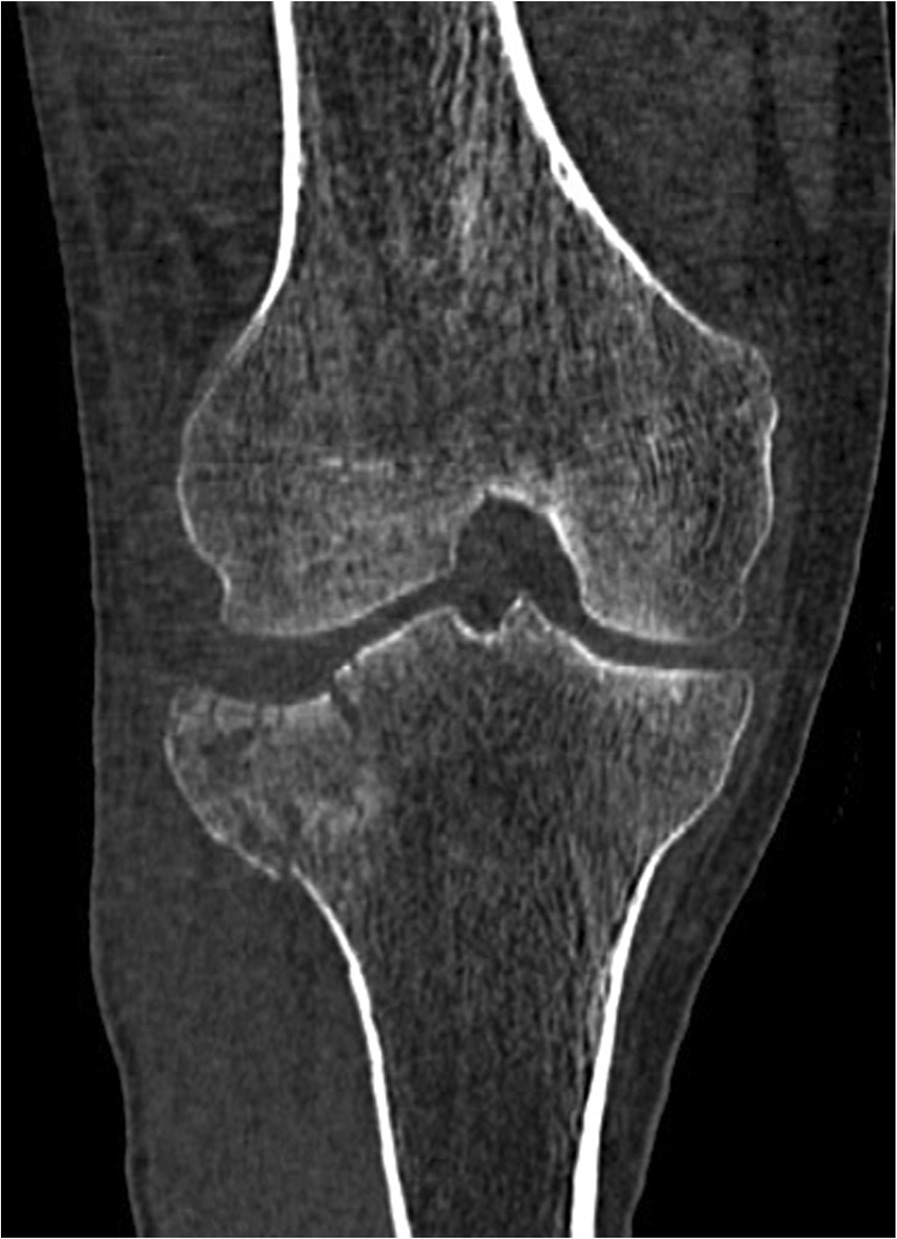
Coronal reconstructions of a computed tomography (CT) scan of the knee showing an AO 41 B3.1 fracture type.
